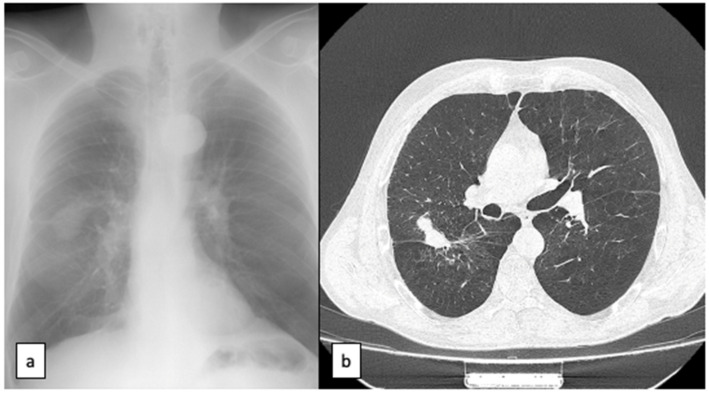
Control after 1 year (2022): (a) chest radiography; and (b) CT scan showing resorption of hematoma with chronicization of lesion, lack of other lung lesions—important remaniation.
